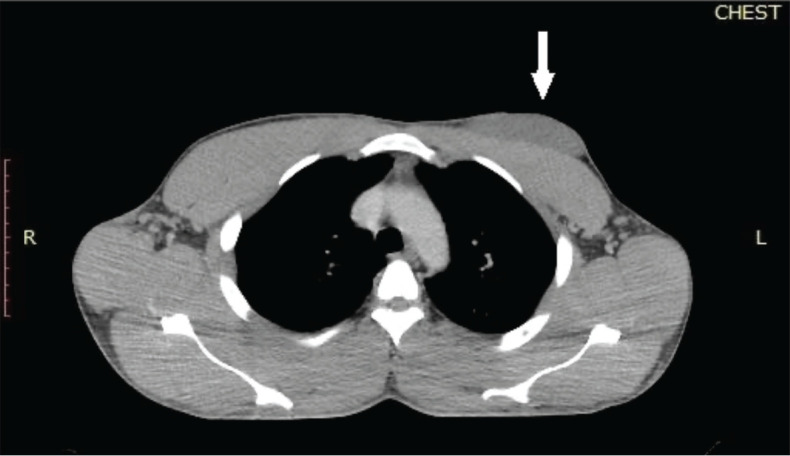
Chest CT scan of the patient which was done after 3 months of systemic treatment. The chest window of a CT scan of the patient showed a hypodense mass located anteriorly to the pectoralis major muscle. The white arrow indicates the residual left breast lesion which was abutting but not infiltrating the underlying chest wall muscle. The size of the tumour was 8.4 × 8.0 × 5.4 cm. The tumour extended from the left parasternal line to the left anterior axillary line.
